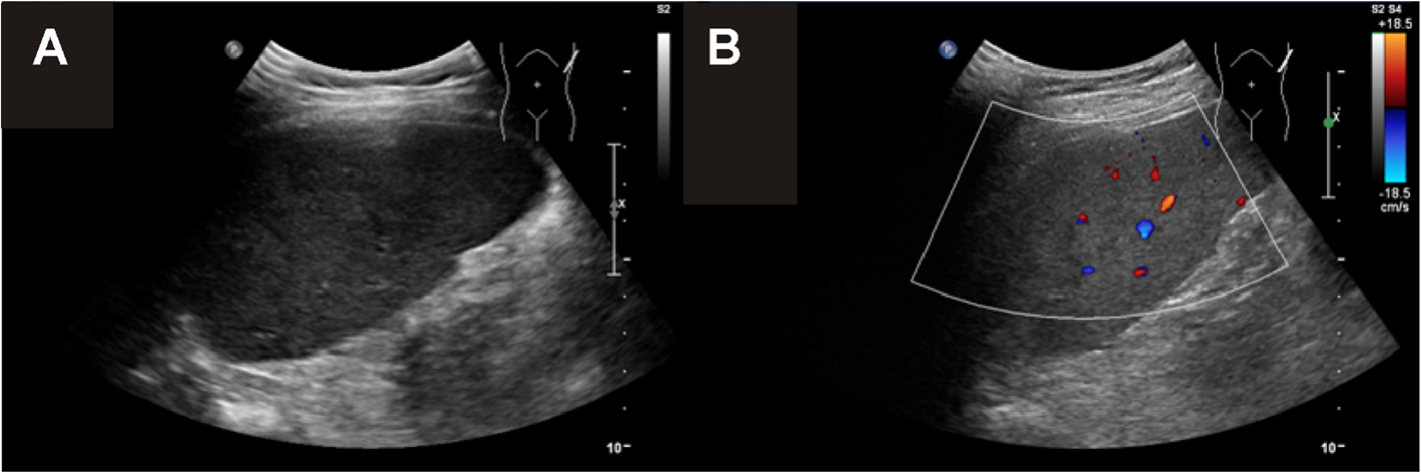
Ultrasound of the spleen after 6 weeks of glucocorticoid treatment. A, B. Follow up B-mode (A) and duplex (B) ultrasound of the spleen show a homogenous texture and normal size.
